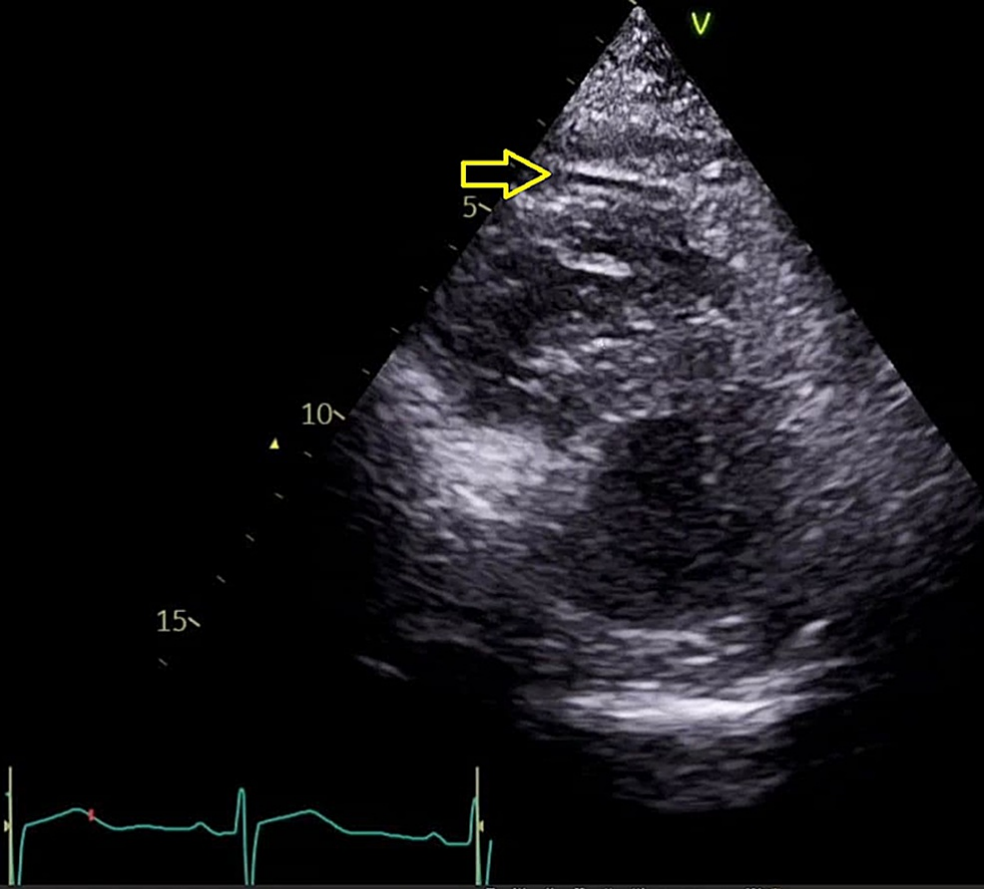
Follow-up transthoracic echocardiography (parasternal short axis view) showing minimal pericardial effusion denoting improvement (April 2021)
